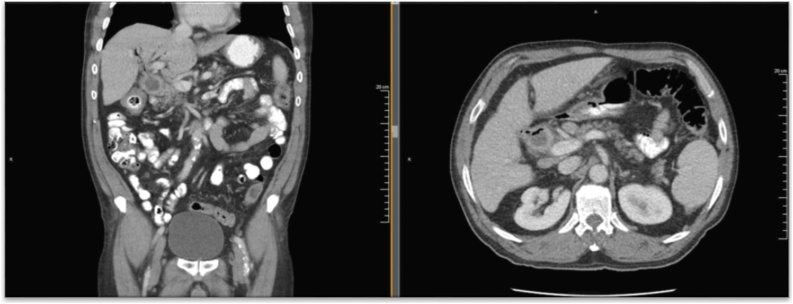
a. coronal section of abdominal CT scan demonstrating thickening extending from the gallbladder to the hepatic flexure of the colon b. transverse section of findings described in 1a.
